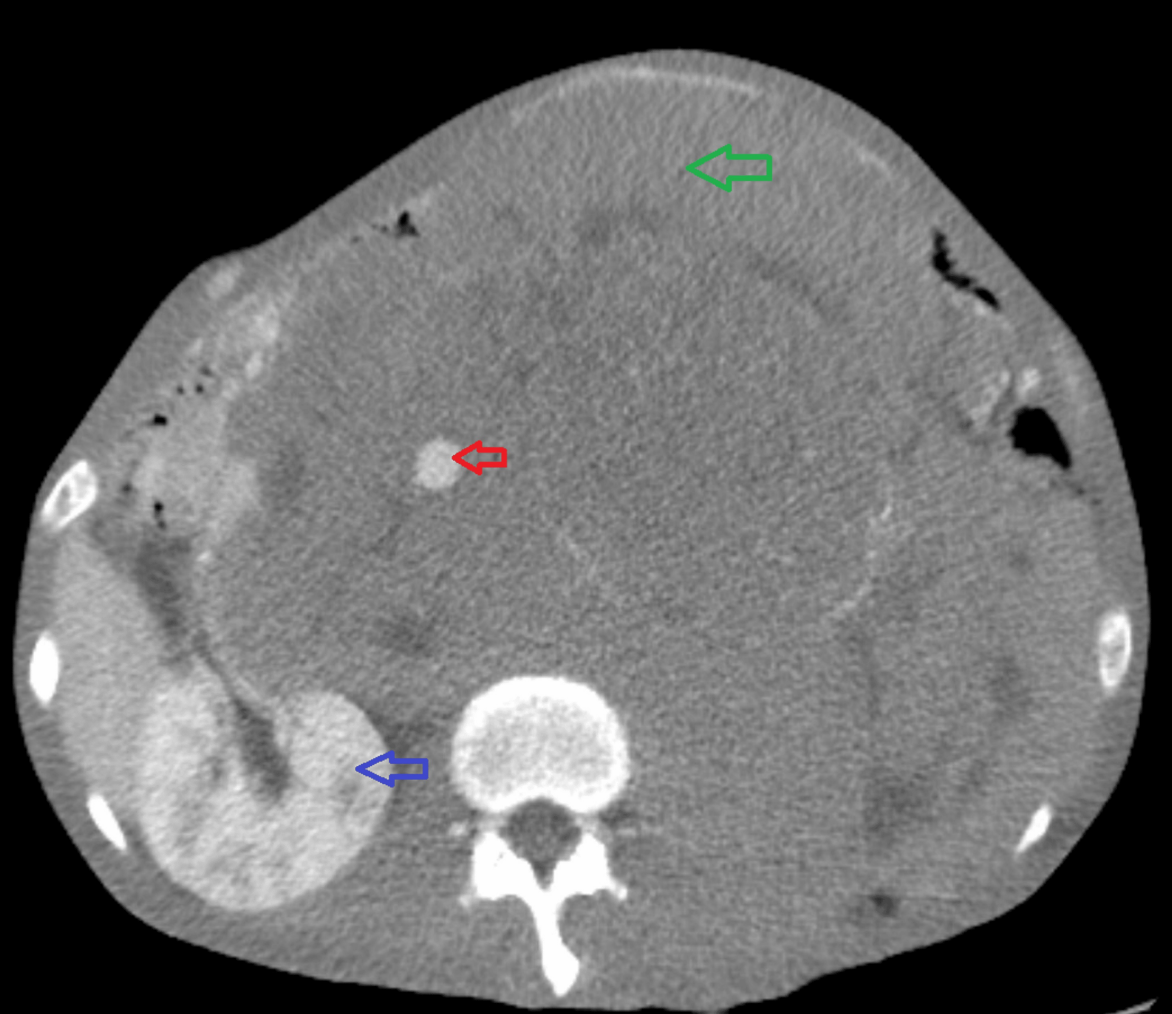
CT of the abdomen and pelvis demonstrating a large heterogeneous intra-abdominal mass (green arrow) displacing the abdominal aorta (red arrow) as well as the right kidney (blue arrow). The left kidney could not be visualized on imaging.
